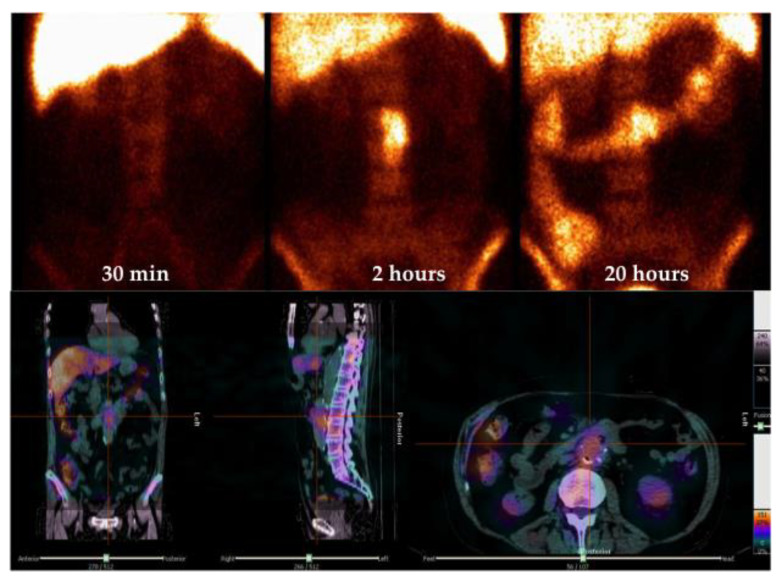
An example of ^99m^Tc-labeled white blood cell (WBC) scintigraphy acquired with times corrected for isotope decay at 30 min, 2 and 20 h p.i. in a patient with suspected abdominal vascular graft infection (VGI). Planar anterior images show an increased uptake in abdominal region that was consistent for an infection (upper panel). Dingle-photon emission computed tomography (SPECT)/CT images (bottom) acquired 2 h p.i. allowed the correct localization of the uptake in the inner of abdominal aortic graft.
